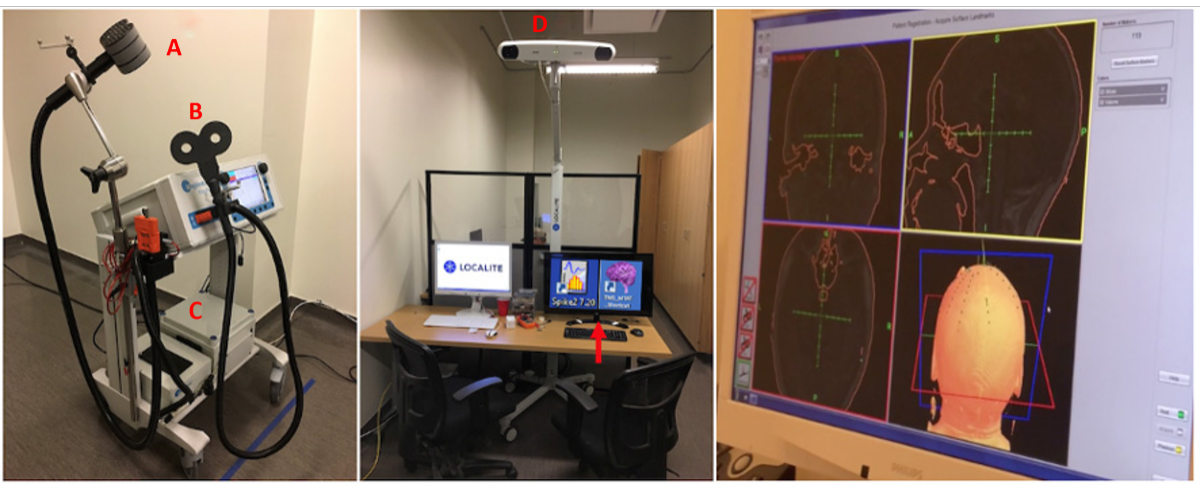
Transcranial magnetic intervention system set-up for the pilot randomized controlled trial. Left: (A) the continuous theta burst stimulation is administered with a MagVenture Cool-B65 stimulator that included an active and sham side; (B) the resting motor threshold was determined using a MagPro X100 stimulator with a figure-of-8 coil; (C) the transcranial magnetic stimulation system is maintained at a constant temperature using an active cooling system. Middle: the stimulation is directed by a computerized system that detected the orientation of the head in space using (D) an antenna camera and correlate it with the individual’s coregistered magnetic resonance imaging scan. Right: An image showing the Neuronavigation system used to administer the transcranial magnetic stimulation to the precise default mode network locations.
